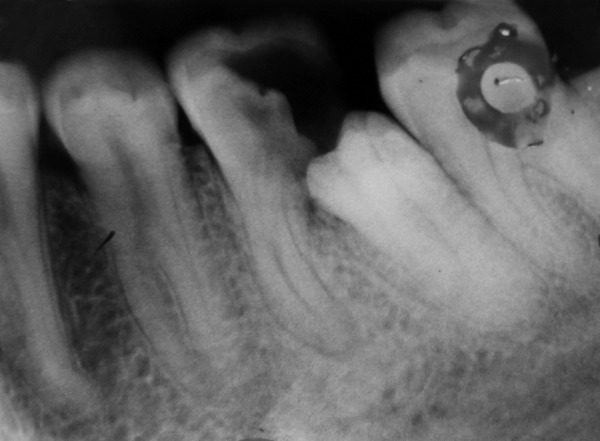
Intraoral radiograph showing paramolar (left side)
